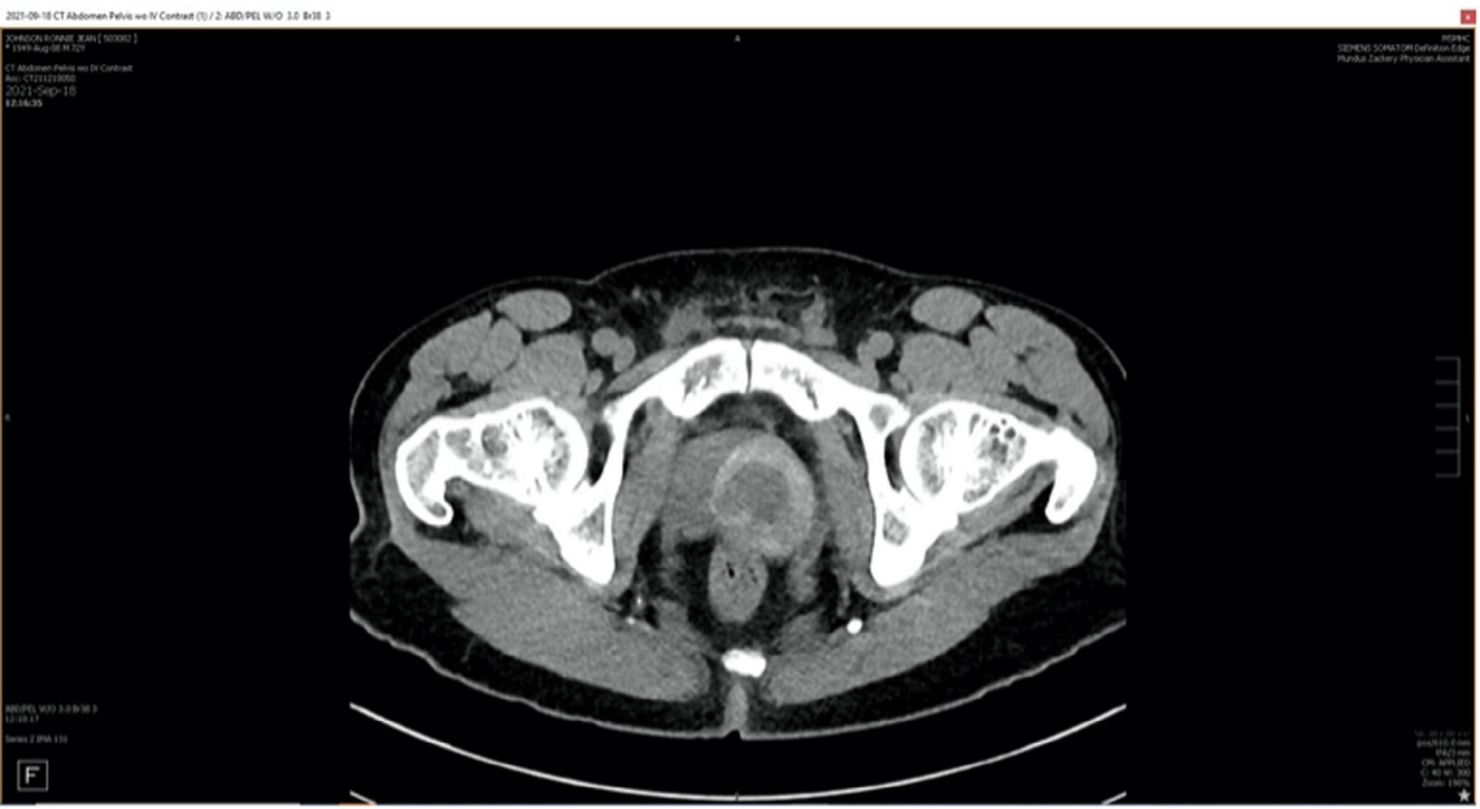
Transverse CT of the abdomen and pelvis. This image depicts a left ureteral kidney stone causing subsequent hydronephrosis of the left kidney. Additionally, a left prostatic mass can be visualized.
